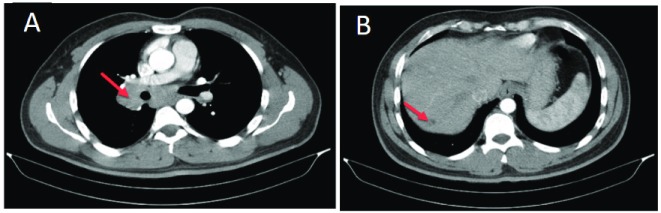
CT scan in-patient with sarcoidosis-(
